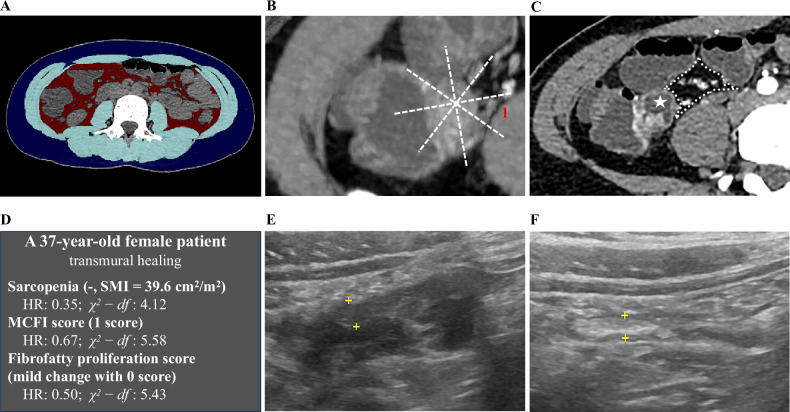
A patient who achieved TH (A–F). A Axial non-contrast CT image at the L3 level shows the segmentation of skeletal muscle (light blue), visceral adipose tissue (red), and subcutaneous adipose tissue (dark blue). The patient was diagnosed with no sarcopenia, with an SMI of 39.6 cm^2^/m^2^. B, C The MCFI and fibrofatty proliferation score of the terminal ileum (indicated by the star) are scored as 1 and 0, respectively. E Pretreatment ultrasonography shows inflammation of the terminal ileum (plus sign). F After 60 weeks of UST treatment, this patient achieved TH, with BWT ≤ 3 mm, normalization of blood flow signal, absence of bowel wall stratification, and no inflammatory mesenteric fat. TH, transmural healing; L3, third lumbar vertebra; MCFI, mesenteric creeping fat index; SMI, skeletal muscle index; UST, ustekinumab; BWT, bowel wall thickness
